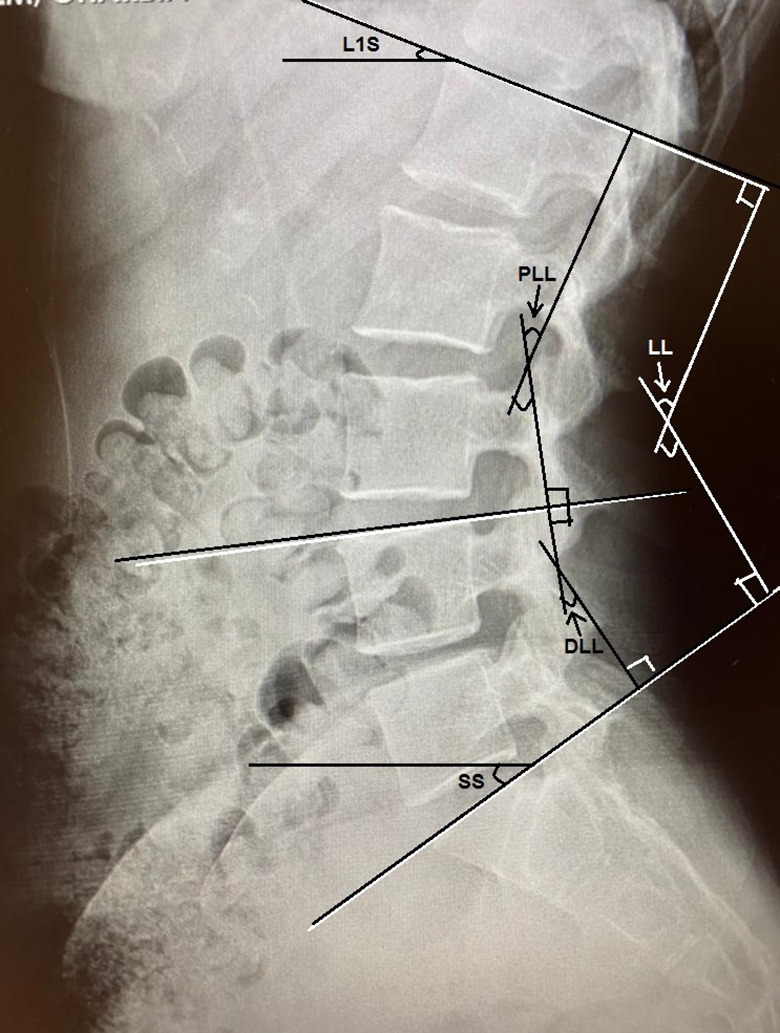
Spinal parameters measured on lateral standing lumbosacral radiography. SS: Sacral Slope, L1S: L1 Slope, LL: Lumbar Lordosis, PLL: Proximal Lumbar Lordosis, DLL: Distal Lumbar Lordosis
